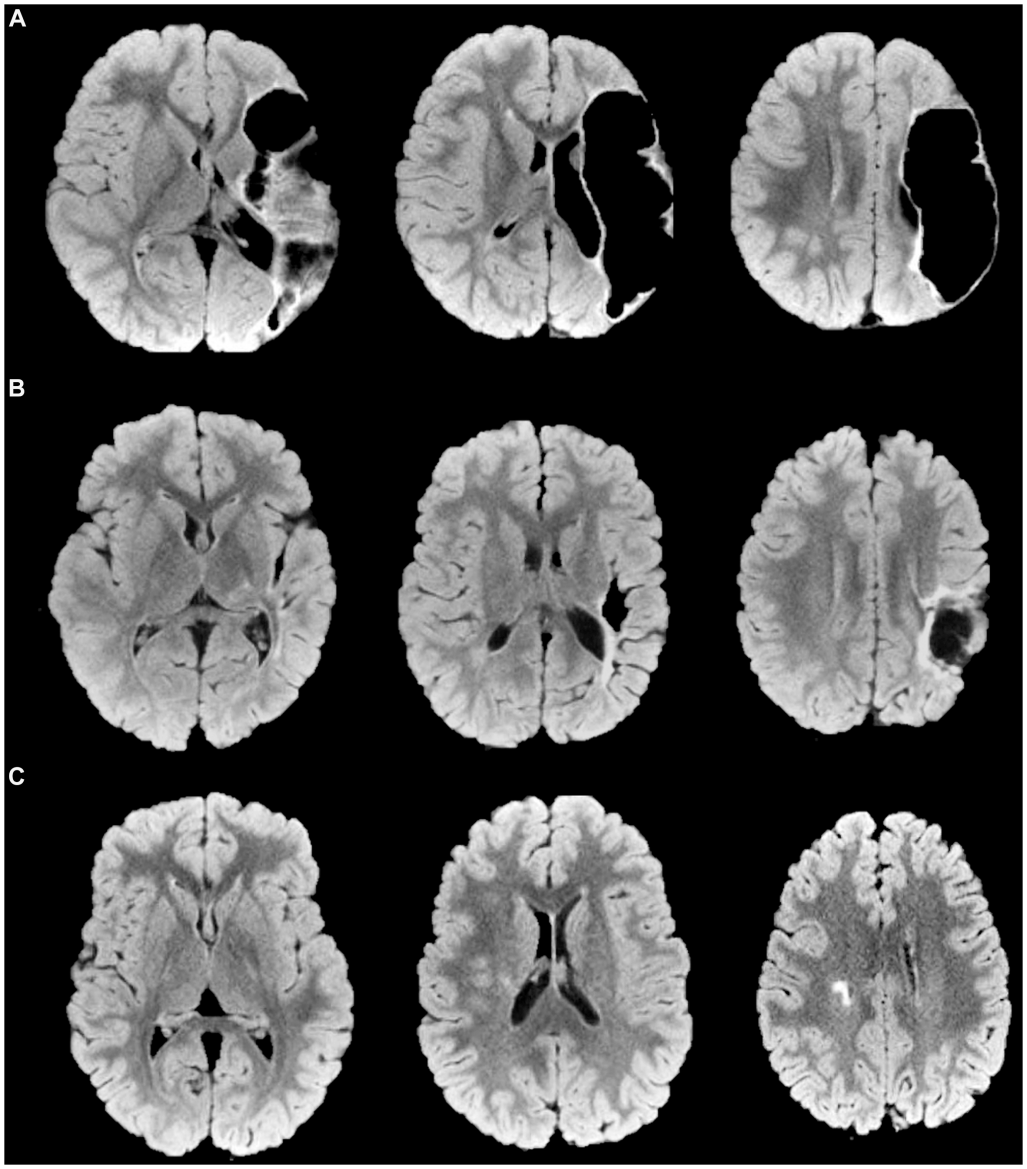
Magnetic Resonance Imaging axial FLuid-Attenuated Inversion Recovery (FLAIR) representative images of some different brain lesion type and severity are provided, with corresponding scores for manual functional impairment and Mirror Movements (MMs). (A) Perinatal stroke in the left middle cerebral artery territory (main branch). MRICS type C, predominant gray matter injury. Brain lesion severity score sqMRI GS: 20.5. More affected hand BBT, 13; MMs, 2. Less affected hand BBT, 48; MMs, 7. (B) Perinatal stroke in the left MCA territory (cortical branch). MRICS type C, predominant gray matter injury. Brain lesion severity score sqMRI GS, 13.5. More affected hand BBT, 48; MMs, 4. Less affected hand BBT, 13; MMs, 1 (C) Right periventricular venous infarction. MRICS B, predominant white matter injury. Brain lesion severity score sqMRI GS, 4. More affected hand BBT, 34; MMs, 2. Less affected hand BBT, 47; MMs, 2. GS, global Score; MRICS, Magnetic Resonance Imaging Classification System; BBT, Box and Block Test; MACS, Manual Ability Classification System; MMs, Mirror Movements.
